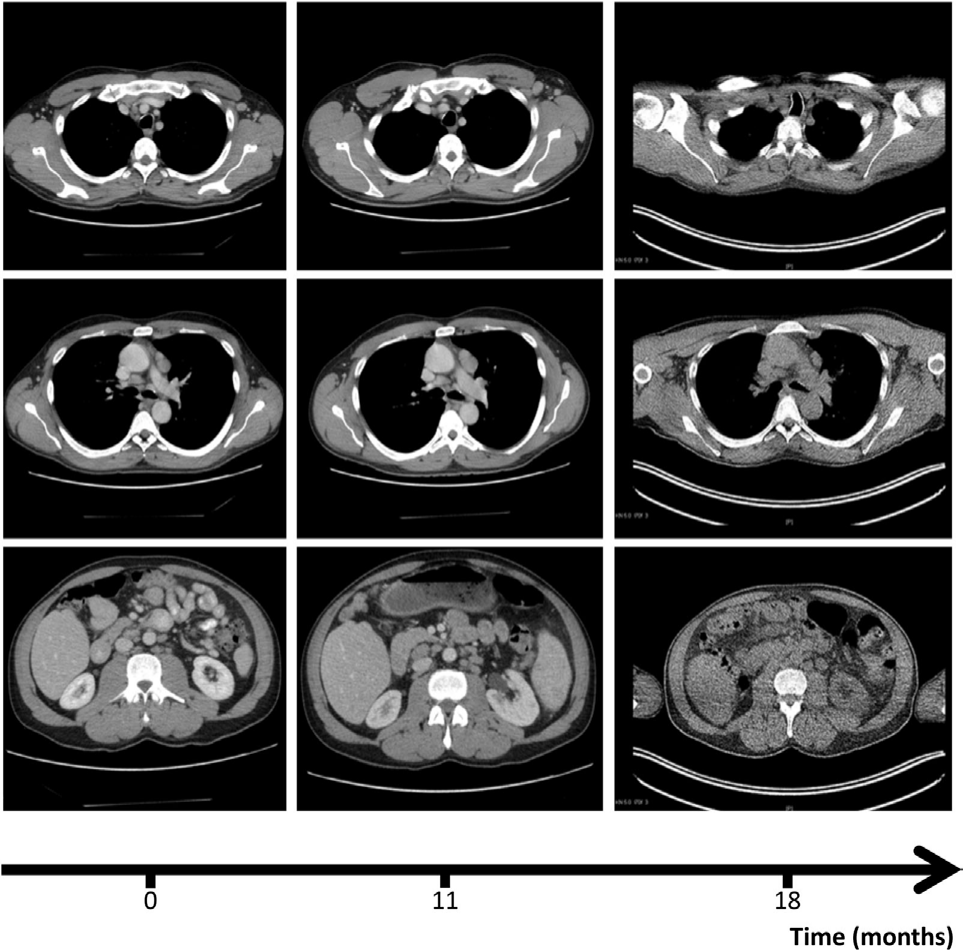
Computer tomography (CT) scans, revealing axillary (upper row), mediastinal (mid row), and abdominal lympadenopathy (lower row) at the three different time points: diagnosis of disseminated infection with Bacillus Calmette–Guérin (0, left column), escalation of antimycobacterial therapy (11, mid column), and diagnosis of Sjögren’s syndrome (18, right column). CT scan of right row was performed in the context of a positron emission tomography/CT (see Figure 4).
